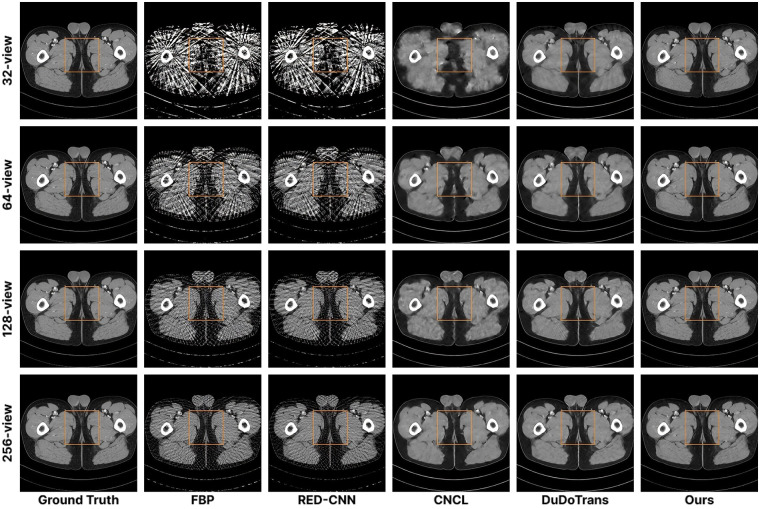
Qualitative comparison on mayo dataset, each row from top to bottom corresponds to 32, 64, 96, 128 views. As shown in the images, the traditional Filtered Back Projection (FBP) method introduces significant stripe artifacts, which are not only visually disruptive but also critically compromise diagnostic accuracy in clinical settings, rendering this approach unsuitable for low-dose CT reconstruction tasks.
